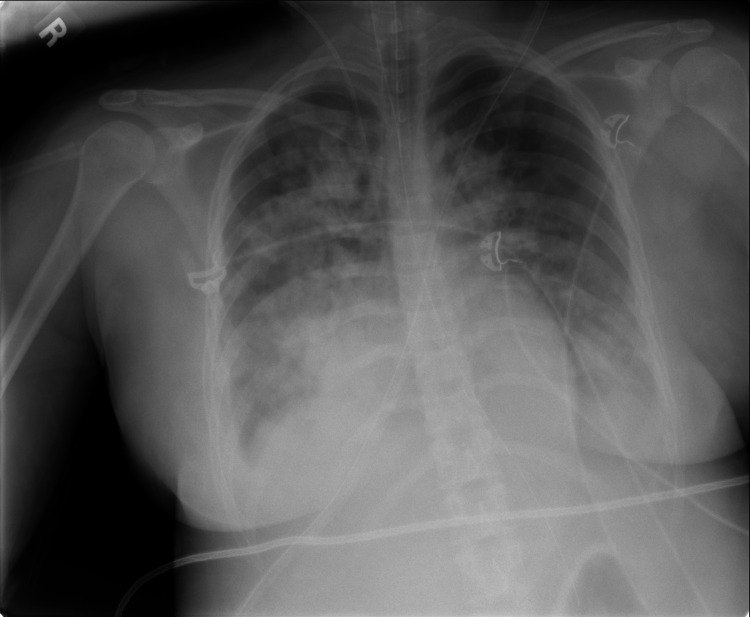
Chest x-ray showing bilateral infiltrates with right-side predominance
